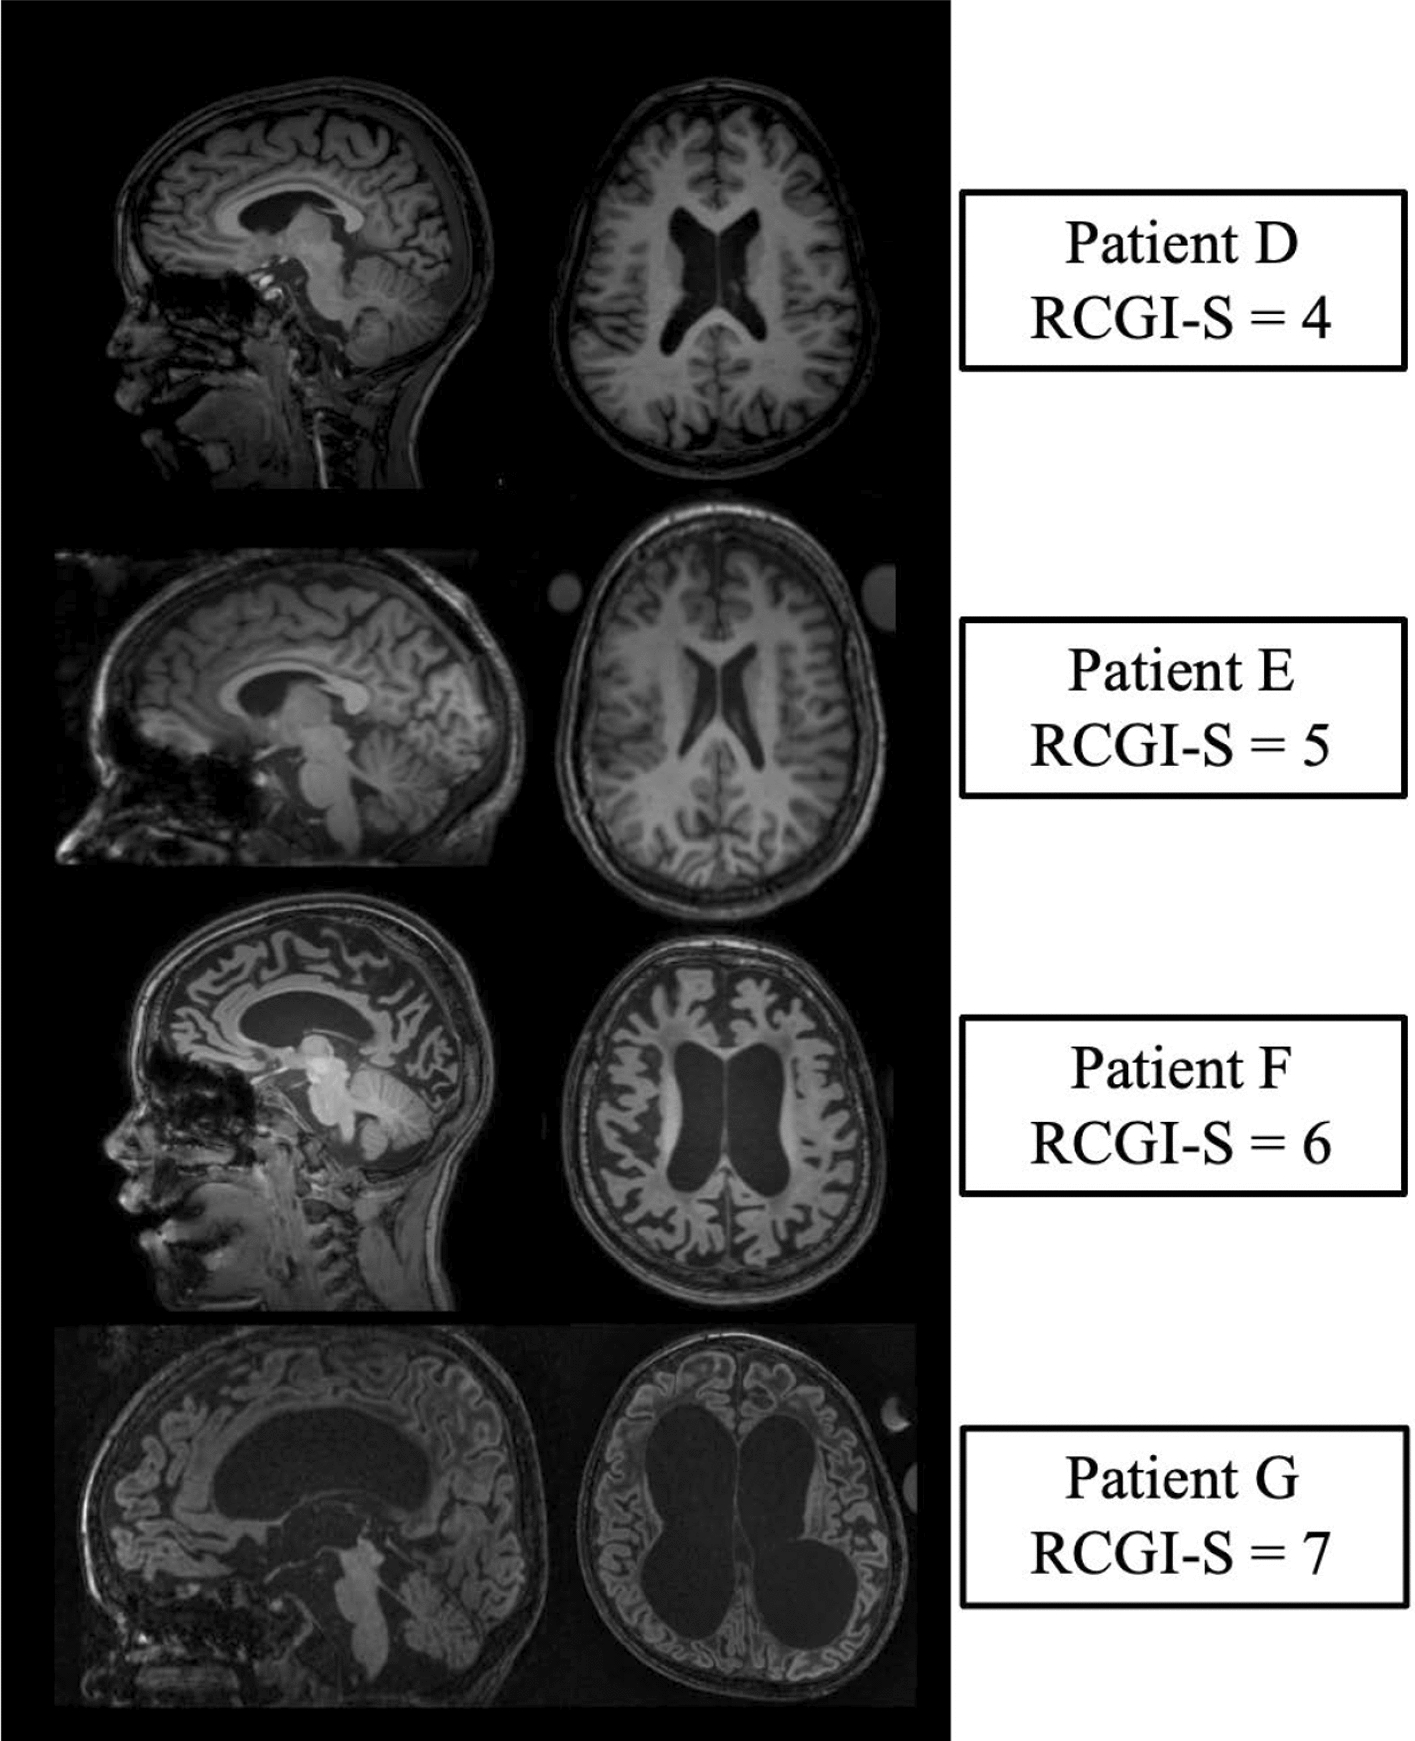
Baseline magnetic resonance imaging for patients D, E, F, and G. Demonstrating worsening atrophy of the corpus callosum and cerebrum, and ventricular enlargement with worsening RCGI-S score
